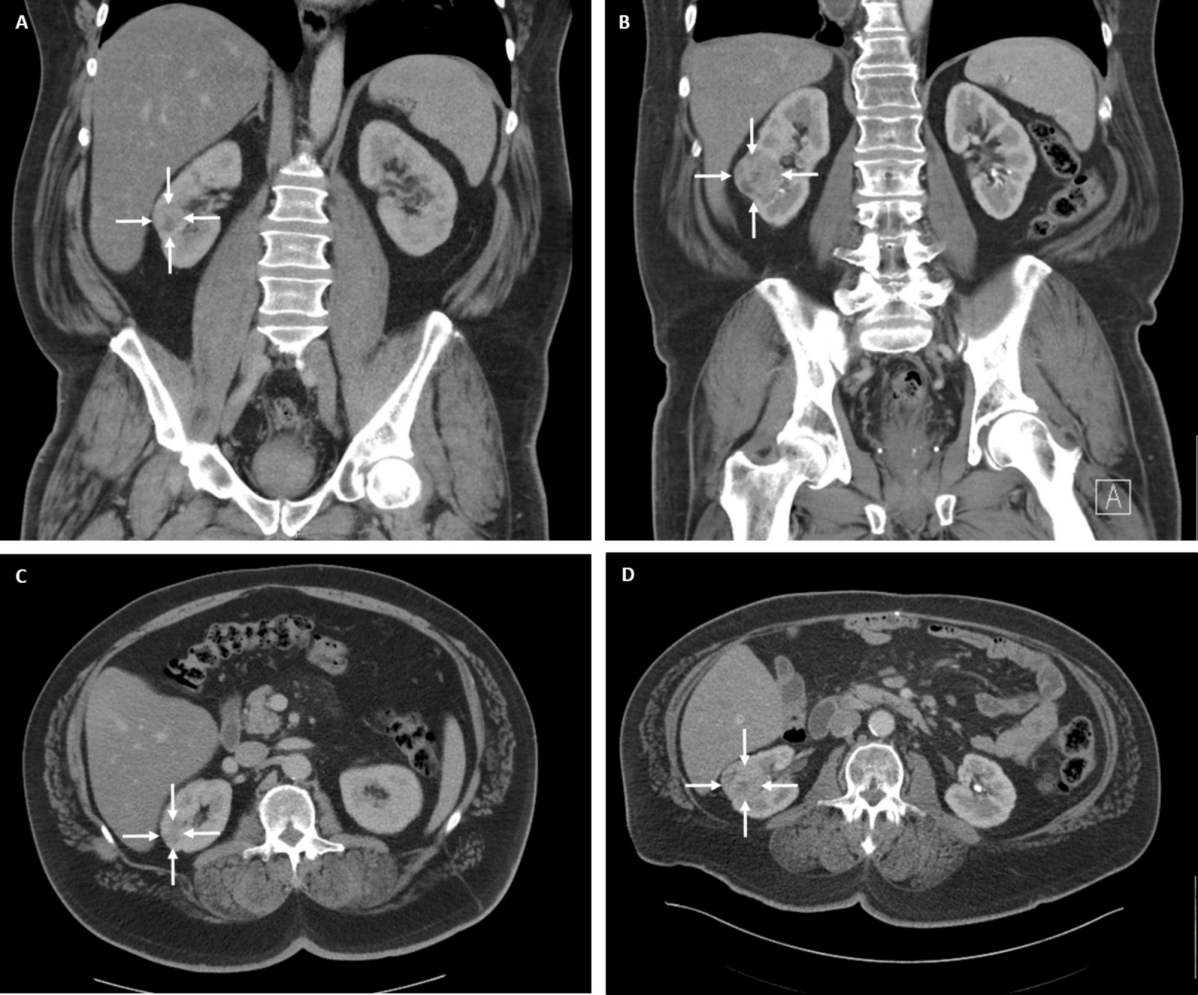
CT imaging of the right renal mass taken two years apart The initial CT coronal (A) and axial (C) views show a solid right renal mass measuring 2.7 cm. Two years later, coronal (B) and axial (D) views show the same solid right renal mass increased in size to 4.1 cm CT: computed tomography
